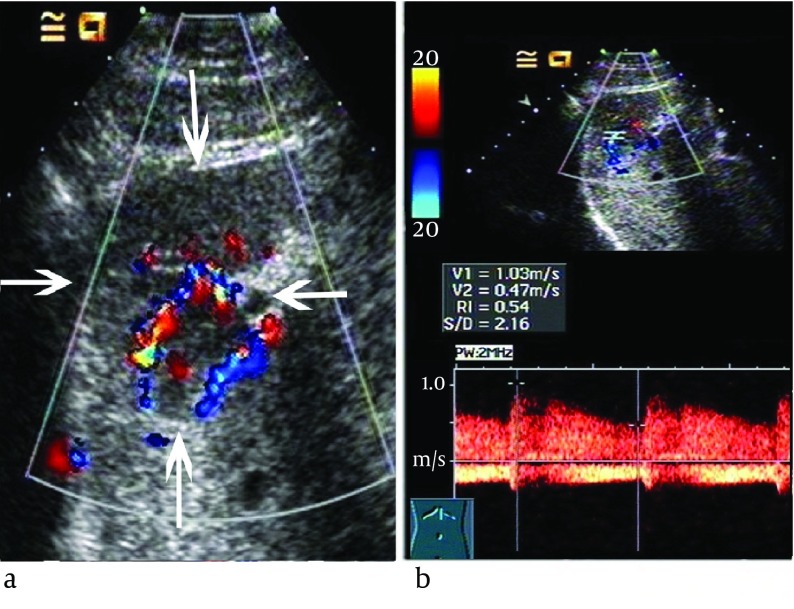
Infantile Hepatic Hemangioendothelioma in a 5-Month-Old Girl (a) Color Doppler revealed plenty of color flow within and adjacent to the nodule (arrows), 2.7 cm in size, in segments 2. (b) Spectral Doppler showed arterial flow with PSV of 103 cm/s and RI of 0.54
